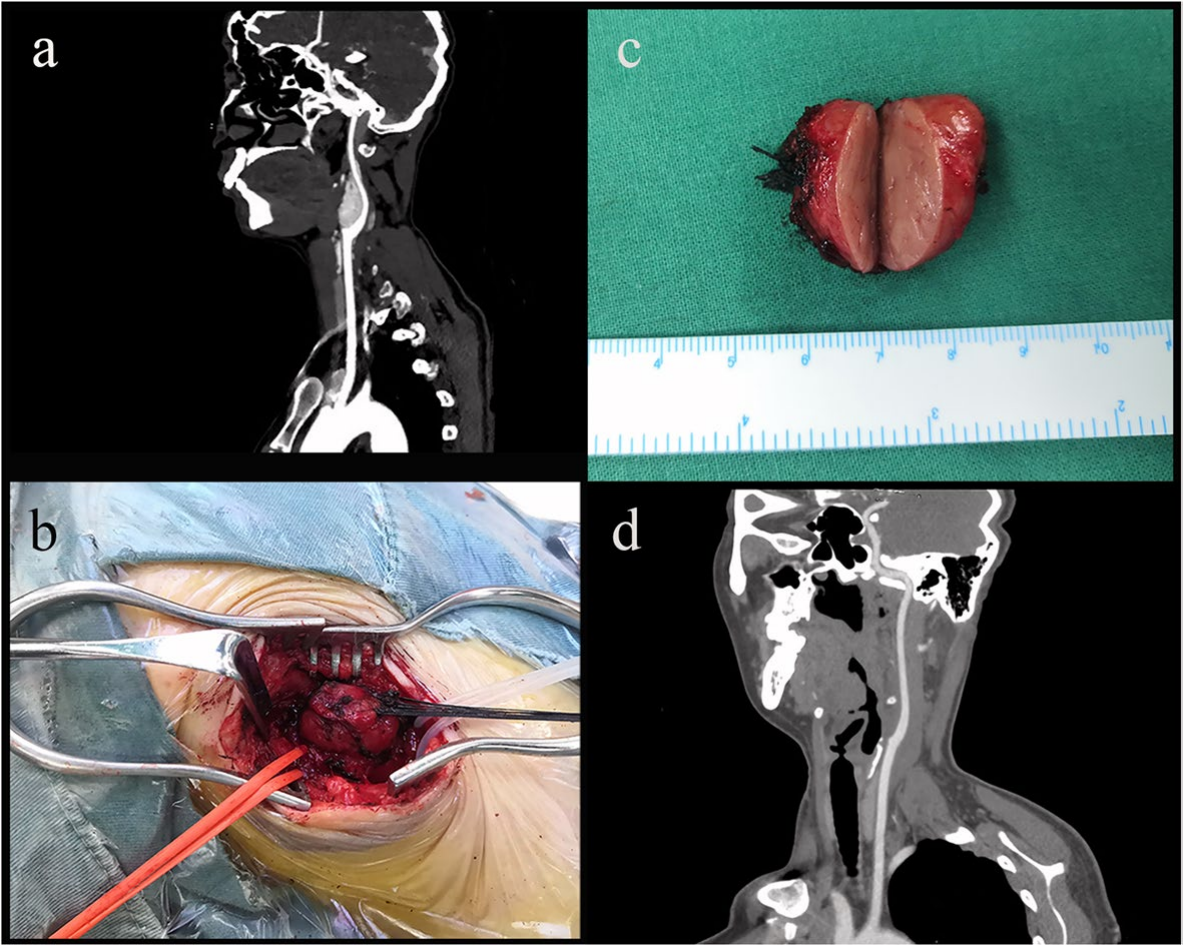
Perioperative images of a CBT patient. a preoperative sagittal CT scan; b exposed CBT with intraoperative control of the arteries; c demonstration of the excised CBT; d postoperative sagittal CT scan
